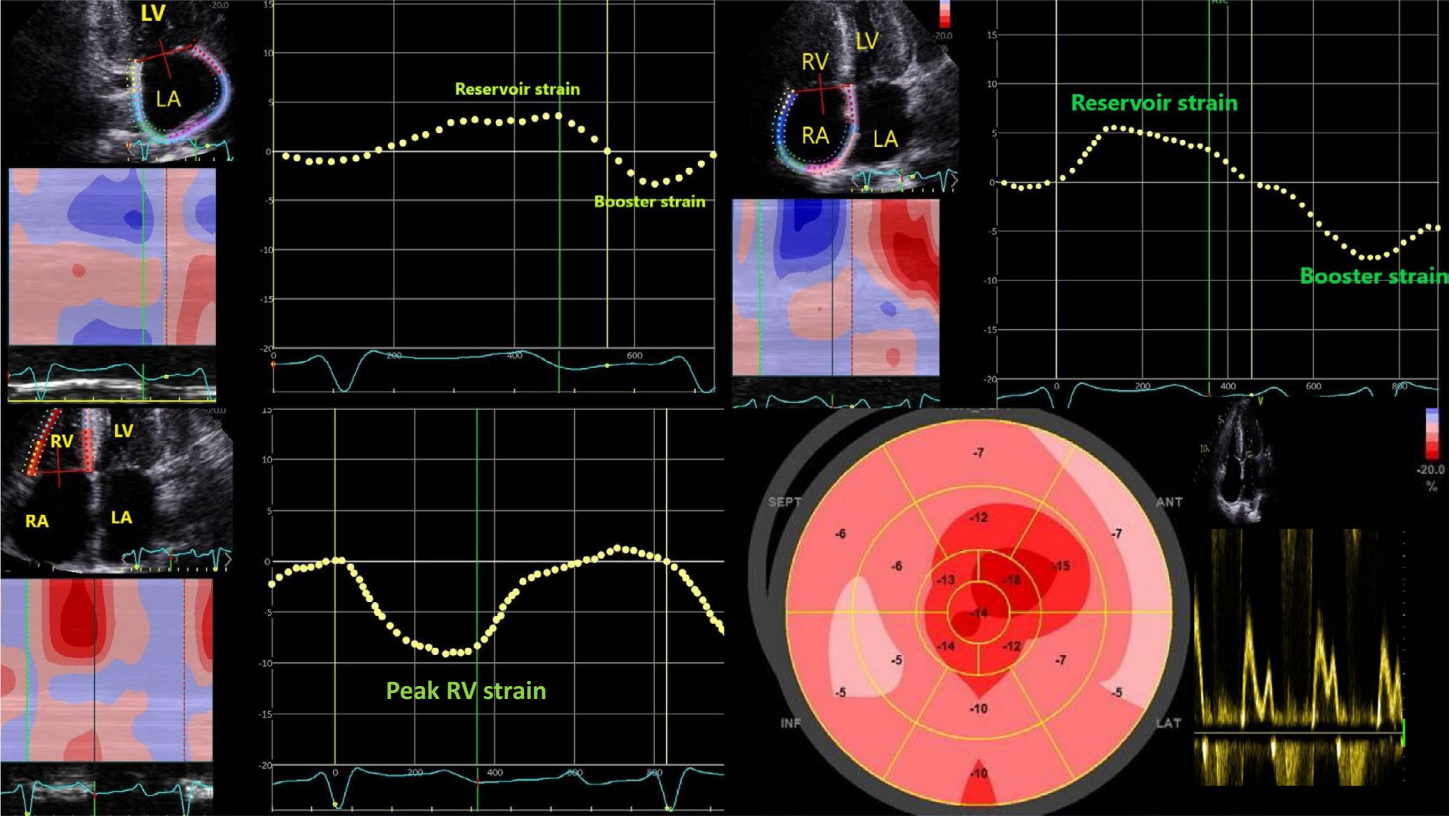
Four‐chamber strain imaging using speckle‐tracking echocardiography in a patient with biopsy‐verified light‐chain amyloidosis. Upper left: atrial (LA) strain showing reservoir and booster components. Upper right: similar phasic strain graphs of the right atrium (RA). Lower left: right ventricular (RV) peak systolic strain. Lower right: left ventricular (LV) global longitudinal strain with a "cherry‐on‐top" strain disposition on Bull's eye pattern of distribution of LV segmental strain and mitral E/A ratio showing pseudonormal LV filling pattern in the same patient
